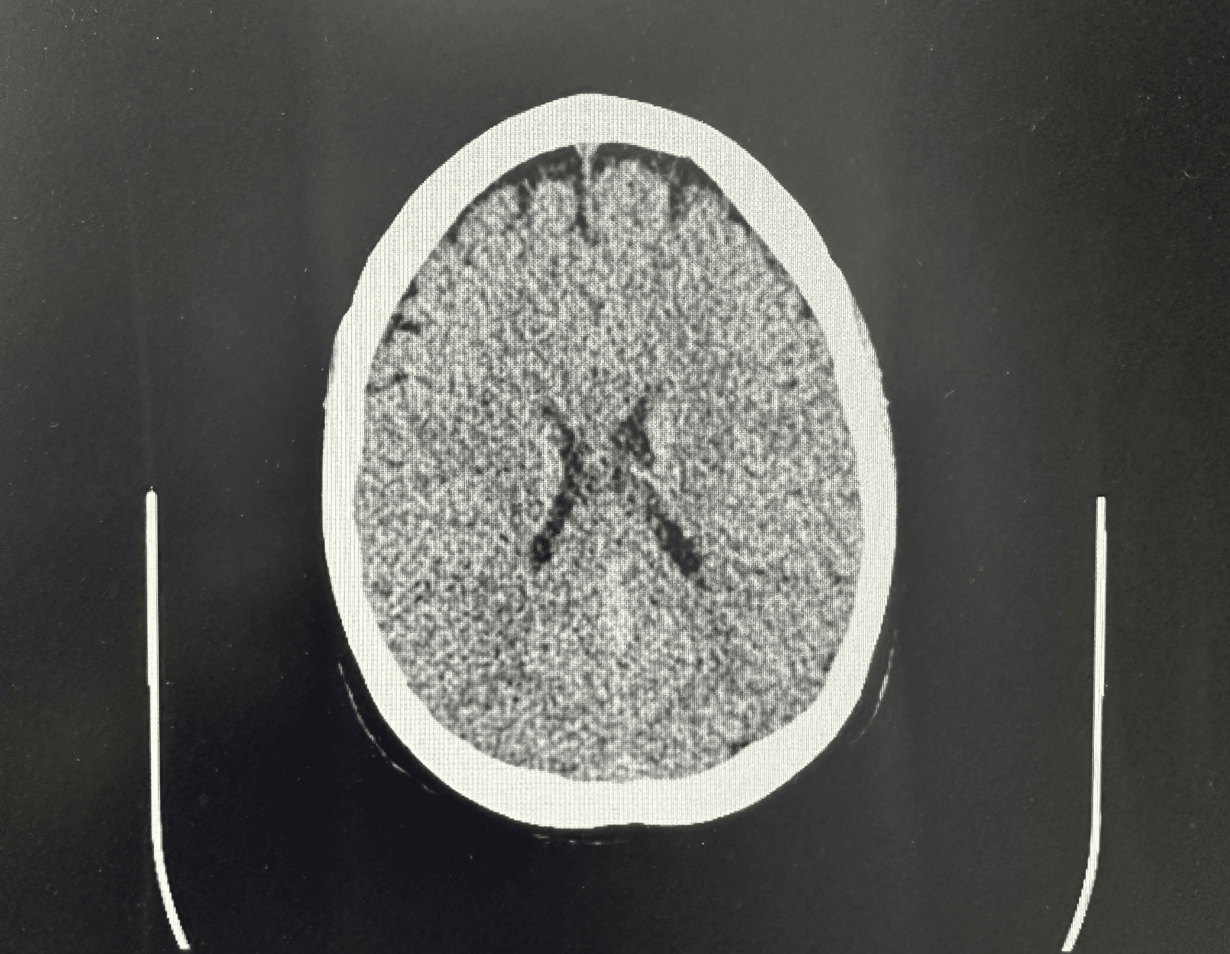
CT axial section showing a normal scan.
